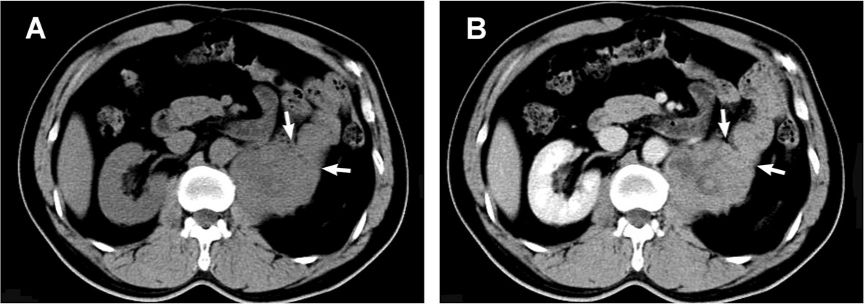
Recurrent mass in the renal fossa. A: CT scanning demonstrates that the tumor attached closely to the psoas muscle and invaded the jejunum (arrow). B: delayed, mild enhancement was prominent in the venous phase.
